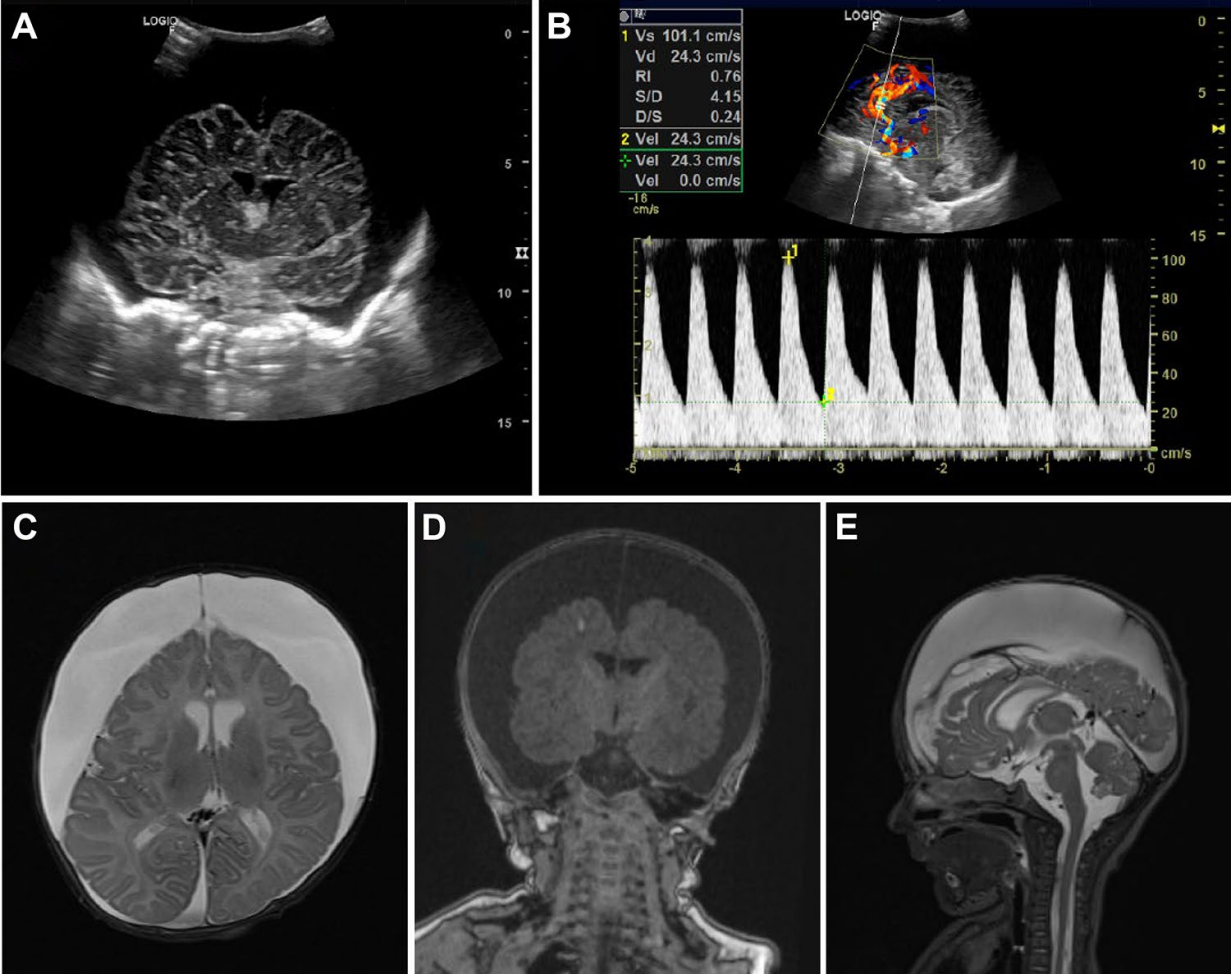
Initial imaging workup after presentation of the patient to the Emergency Department because of macrocephaly, lethargy, and vomiting. A Ultrasound scan in the coronal plane, demonstrating extensive bilateral hygroma and B the corresponding transcranial Doppler with a resistance index of 0.76. C Preoperative MRI with T2-weighted sequence in the axial plane, D T1-weighted sequence in the coronal plane, and E T2-weighted sagittal plane basically confirming the ultrasound findings
